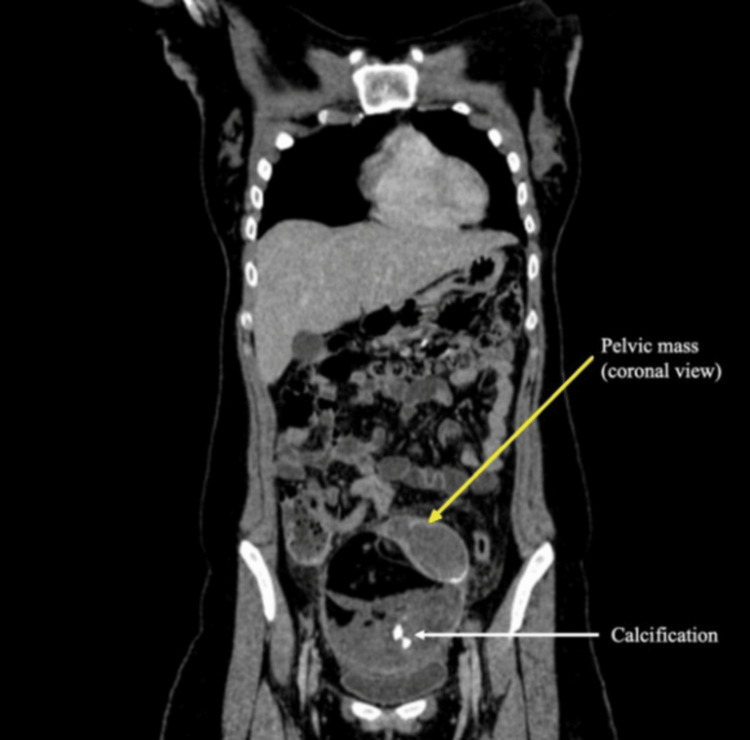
Radiographic contrast-enhanced computed tomography pelvis (coronal section) locating the pelvic mass of size 112 x 98 x 118 mm with area of internal calcification. The yellow arrow indicates the pelvic mass, and the white arrow indicates the calcification.
